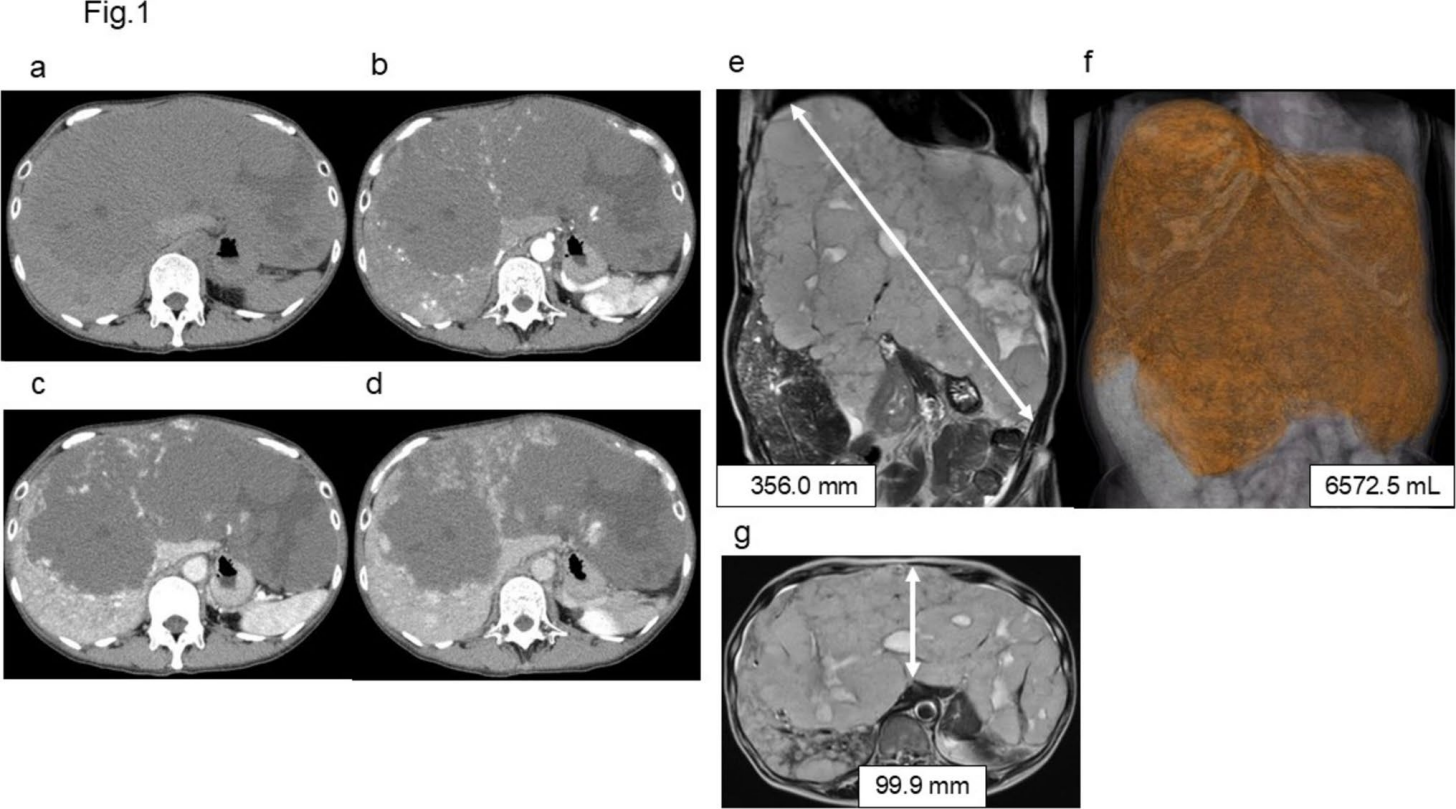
Initial contrast-enhanced CT of the giant hemangioma obtained at our hospital (a–d). a Plain image. b Hepatic arterial phase. c Portal venous phase. d Delayed phase. T2-weighted MRI shows the giant hemangioma immediately before initiation of sirolimus treatment (e–g). e The maximum diameter is 356.0 mm. f The volume is 6572.5 mL. g The distance to the anterior abdominal midline is 99.9 mm
